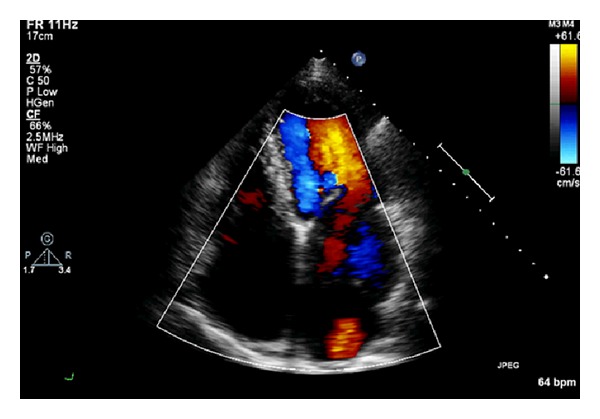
Two-dimensional echocardiographic view (apical 4 chamber view) showing jet of mitral regurgitation.
